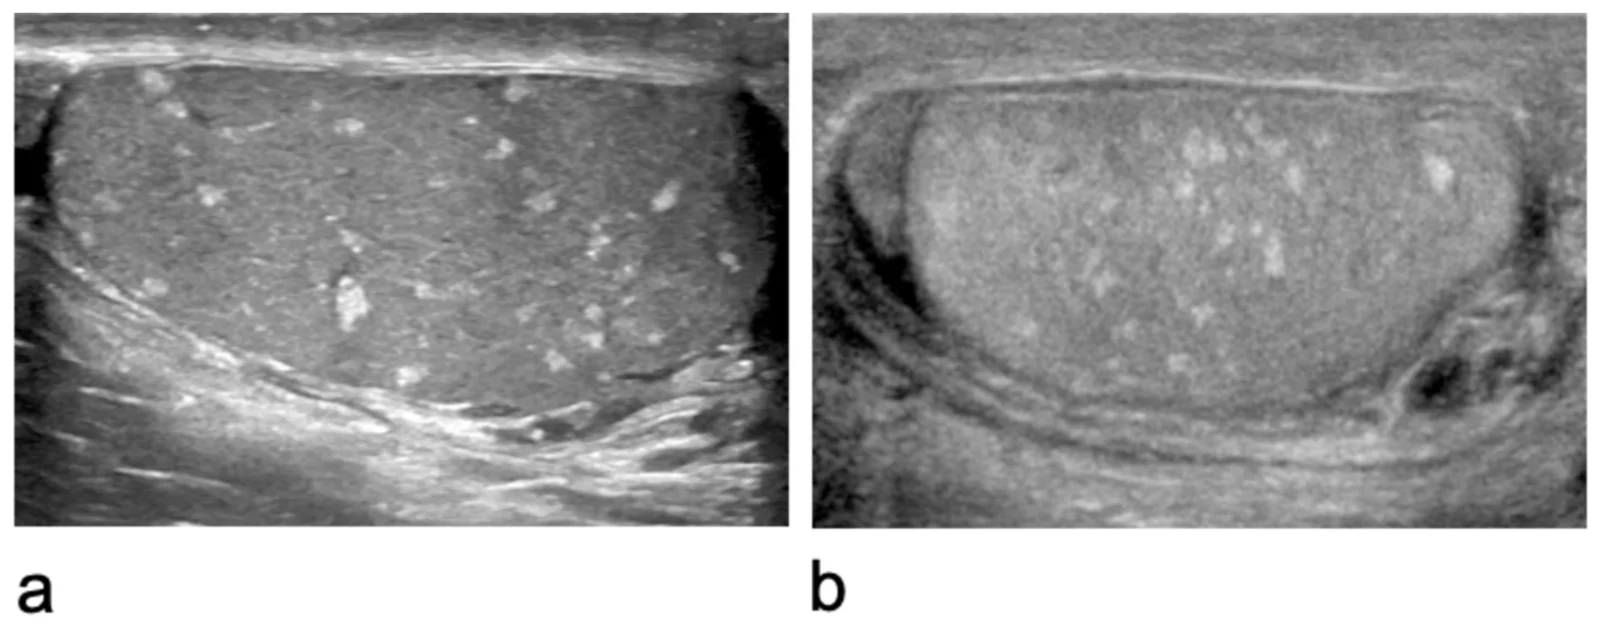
Two different patients (a,b) underwent scrotal ultrasound for unrelated indications. In both cases, ultrasound showed multiple bilateral hyperechoic intratesticular lesions. The diagnosis of Cowden syndrome was confirmed by diagnostic testing in patient (a), whereas testing was negative in patient (b).
